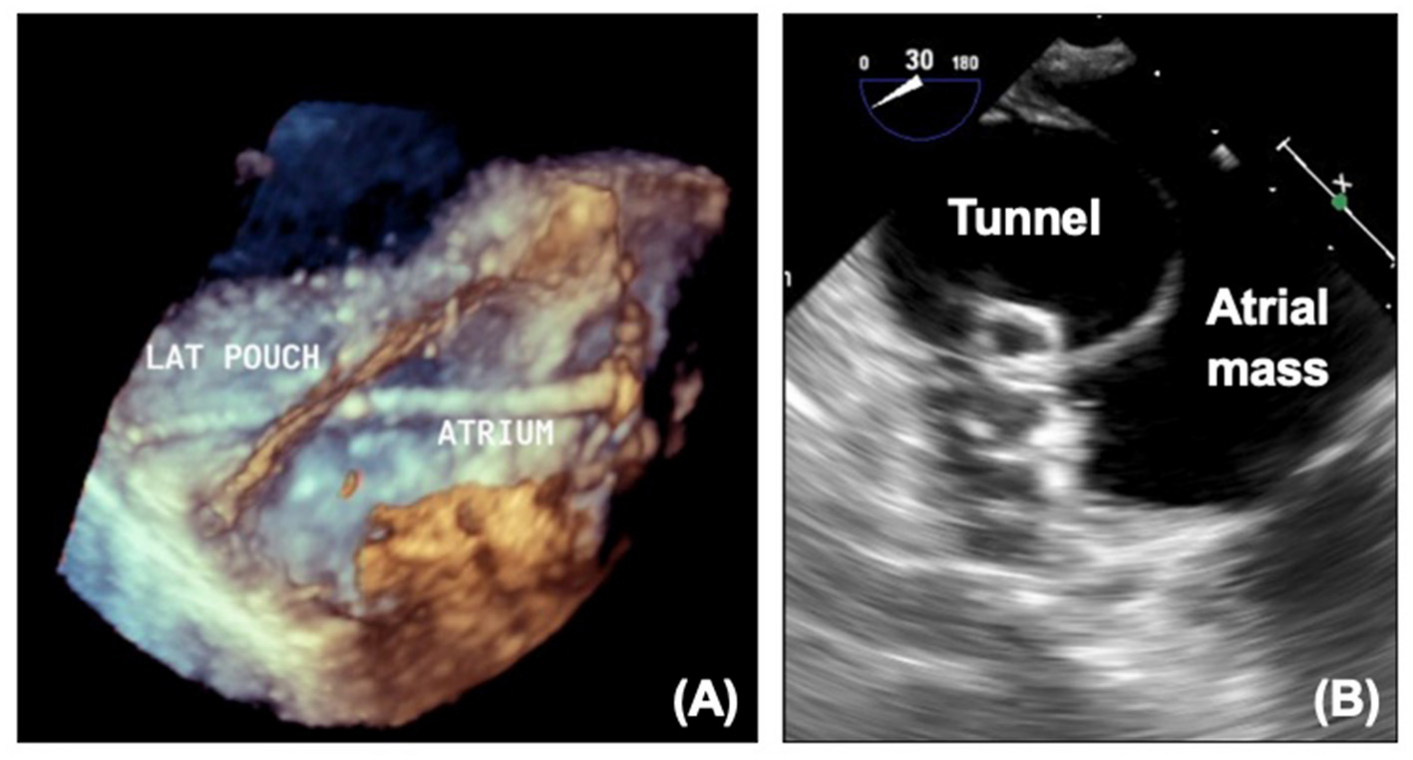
(A) 3D echocardiogram showing wire across fenestration. (B) Device in fenestration seen on echocardiography. LAT, Lateral tunnel pouch.
